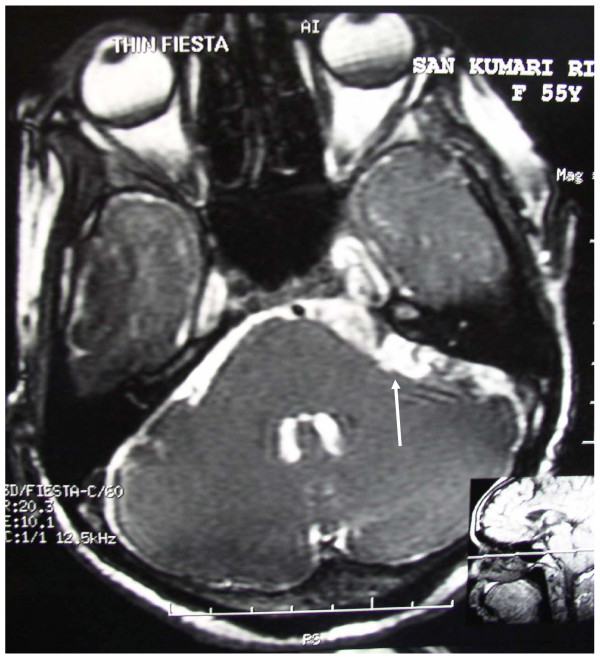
MRI (FIESTA) showing the left CP angle epidermoid (arrow) involving the Vth nerve and Gasserian ganglion.
